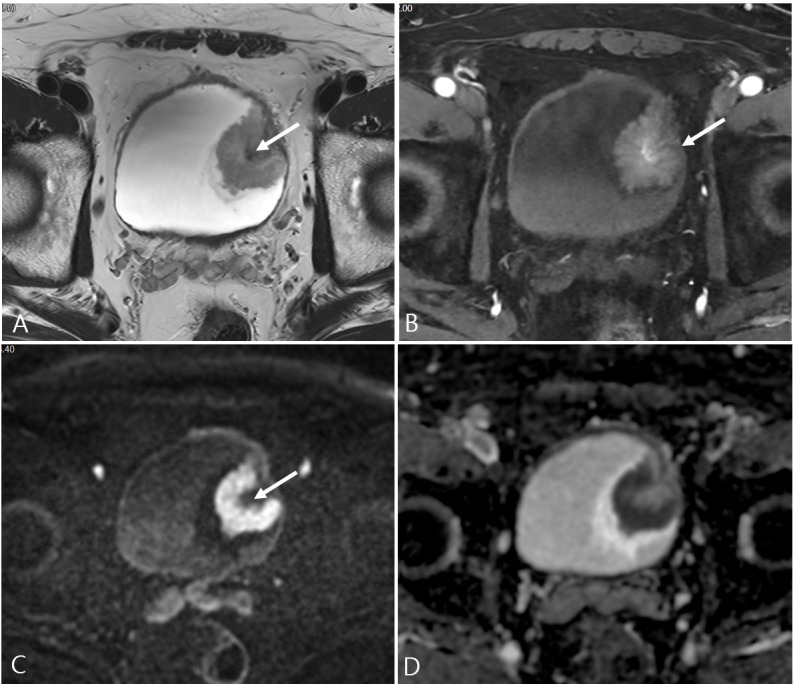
VI-RADS 2—Non-muscle-invasive bladder cancer. A 69-year-old male presenting with a 4.3-cm papillary mass in the left lateral wall of the bladder. (A) Axial T2-weighted imaging (T2WI) demonstrates a large papillary mass with an intact low-signal muscularis propria, showing a tumor stalk sign (arrow) and a suspected thickened inner layer, consistent with the involvement of the urothelium and lamina propria without muscle invasion. (B) Axial T1-weighted contrast-enhanced imaging (T1CE) shows enhancement confined to the tumor body, while the underlying muscularis propria maintains preserved low-signal intensity (arrow), indicating intact muscle layer integrity and supporting non-muscle-invasive disease. (C) High b-value diffusion-weighted imaging (DWI; b = 1000 s/mm^2^) demonstrates marked diffusion restriction within the tumor lesion, sparing the tumor stalk (arrow), which is suggestive of the inchworm sign. (D) The apparent diffusion coefficient (ADC) map confirms significantly reduced ADC values, reflecting high tumor cellularity. The overall imaging features are consistent with a VI-RADS score of 2, suggesting a non-muscle-invasive tumor confined to the mucosa or lamina propria. Subsequent transurethral resection confirmed pathological stage T1 urothelial carcinoma, consistent with the mpMRI-based VI-RADS assessment.
